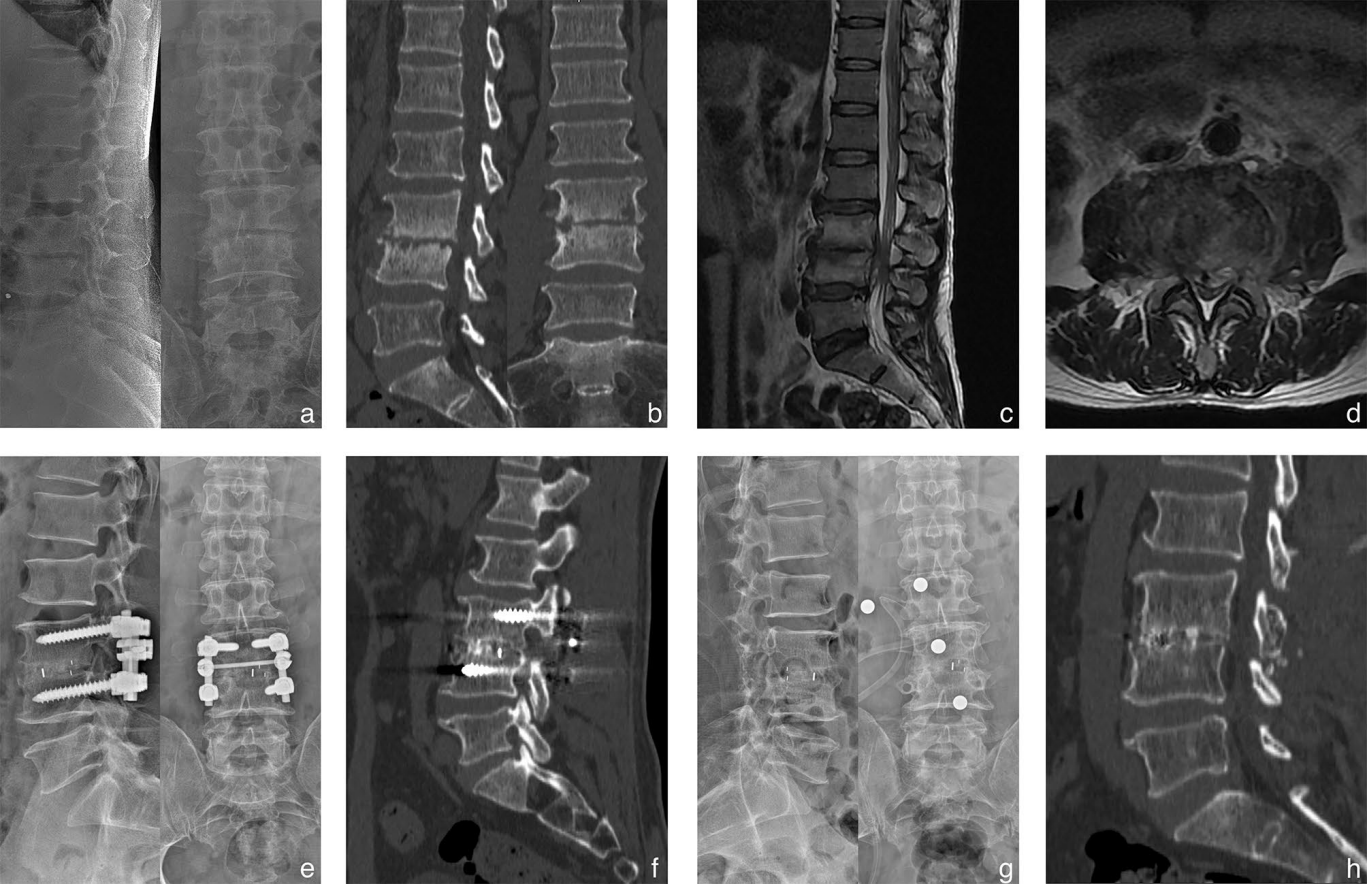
A 53-year-old male shepherd diagnosed with L3-4 brucella spondylitis underwent one-stage posterior interbody fusion and debridement procedure. Preoperative X-ray revealed intervertebral height loss and significant narrowing of the affected disc space. Preoperative CT and MRI scans exhibited erosions of the superior and inferior endplates, along with evidence of nerve compression. (a–d) Postoperative X-ray and CT images demonstrated satisfactory positioning of the internal fixation and cage. (e–f) The final follow-up conducted at 26 months indicated successful bone fusion following the removal of instrumentation, as evidenced by radiographic and CT examinations (g–h).
